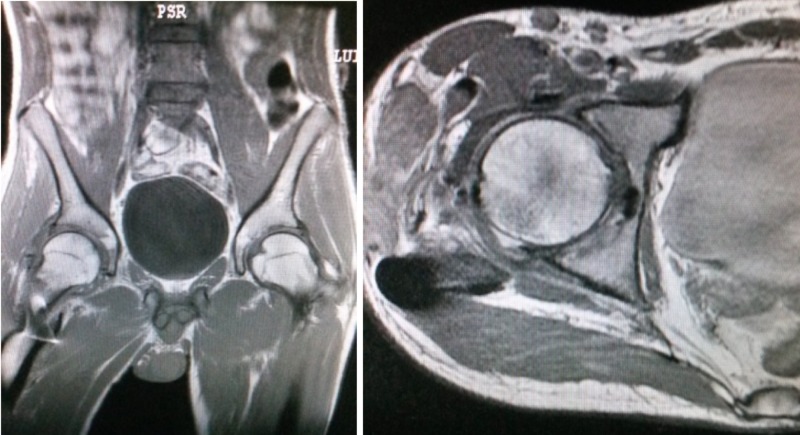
Pictures of the MRI, showing the reduction of the articular space and no necrosis of the femoral head
